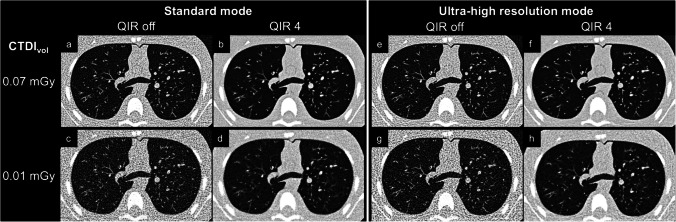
Representative axial images of scans acquired in the standard mode (a-d) and the ultra-high resolution mode (e–h) at the two lowest radiation doses (volume CT dose index, CTDI_vol_) of 0.07 mGy (a, b, e, f) and 0.01 mGy (c, d, g, h). Scans were reconstructed both without using quantum iterative reconstruction (QIR off) (a, c, e, g) and with QIR at strength 4 (b, d, f, h). Note the considerably reduced image noise and augmented visualization of small peripheral structures in reconstructions with QIR 4 (b, d, f, h) compared to those without QIR (a, c, e, g). *CT* computed tomography, *CTDI*_*vol*_ volume CT dose index, *QIR* quantum iterative reconstruction
